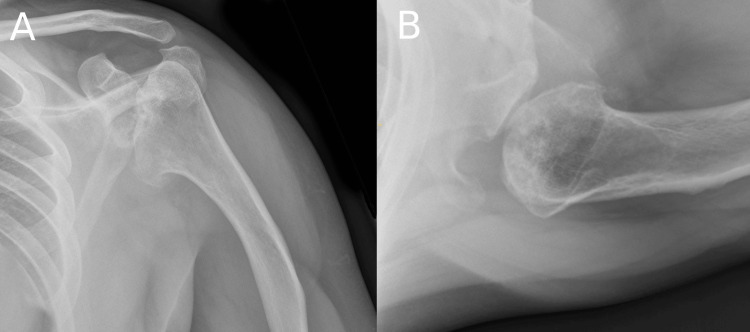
X-rays of the left shoulder at presentation (A) Anteroposterior view; (B) Axial view
